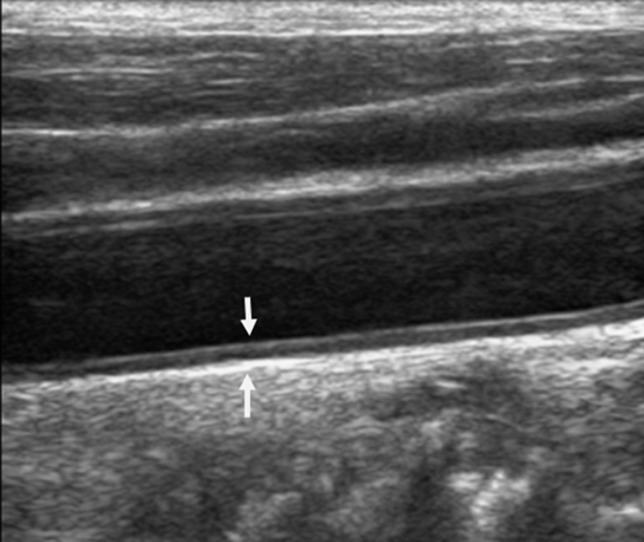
B-mode, longitudinal ultrasound of the carotid artery showing the intima-media thickness in between the white arrows
